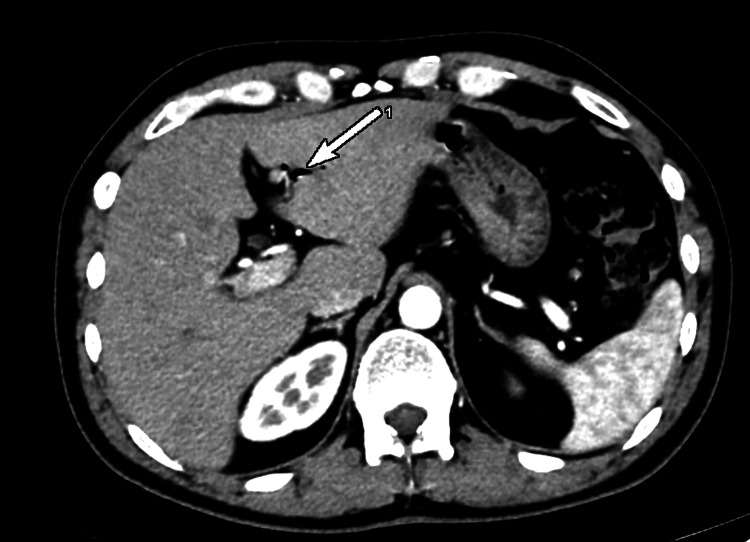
Computed tomography; intrahepatic aerobilia (white arrow).
